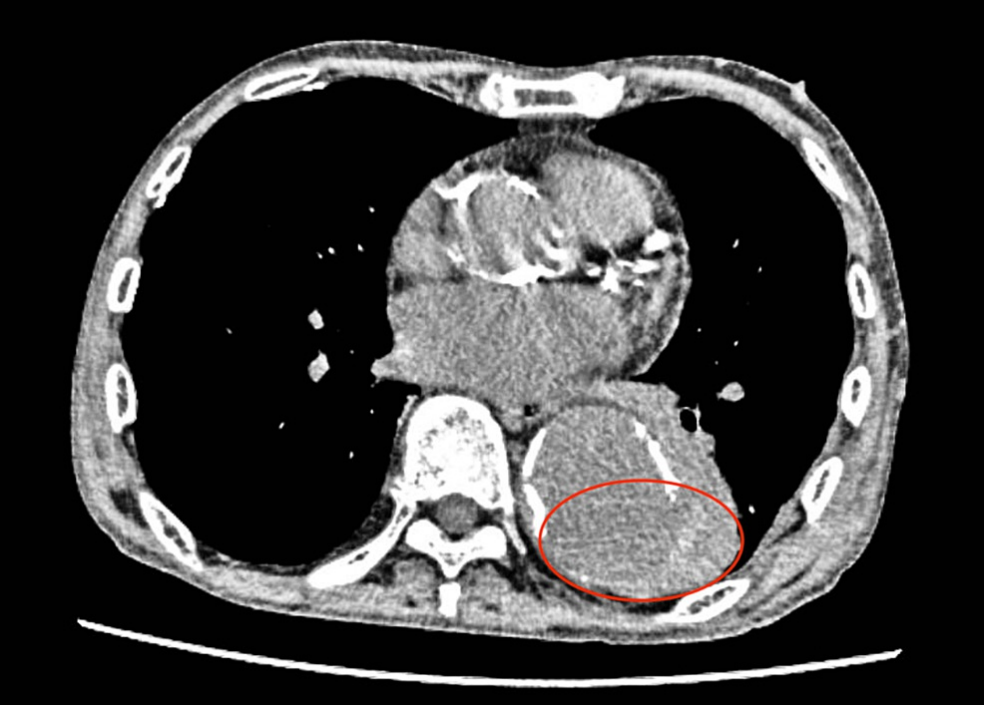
CT findings showing thoracic aortic aneurysm with irregular margins and partial high density (red circle)
